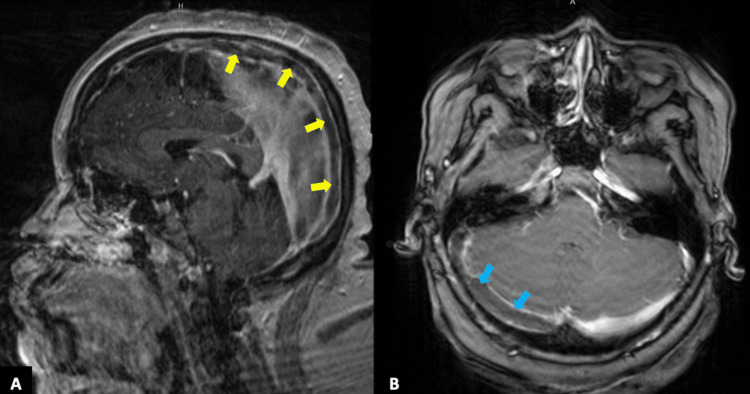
T1-weighted contrast-enhanced MRI of the brain. Sagittal reformatted image (A) demonstrating a filling defect within the superior sagittal signal (yellow arrows) consistent with thrombosis. The axial image (B) demonstrates the extent of the thrombosis with extension into the right transverse sinus (blue arrows).
